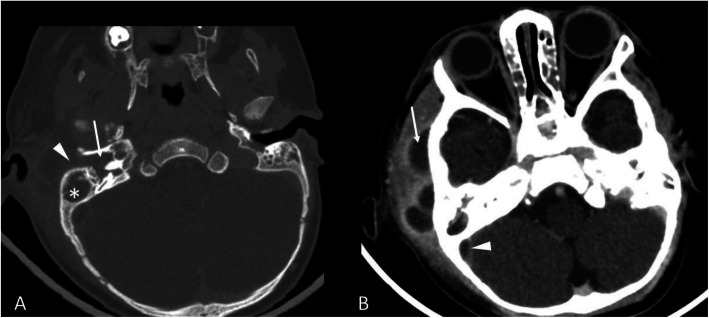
a On bone window of the pre-contrast CT showed complete obliteration of the tympanic cavity (arrow), the mastoid (asterisk) and the external auditory canal (arrowhead), compatible with an otomastoiditis. b Contrast-enhanced CT showed multiple abscesses in the right peri-auricular soft-tissue (arrow) and the thrombosis of the right sigmoid sinus (arrowhead)
